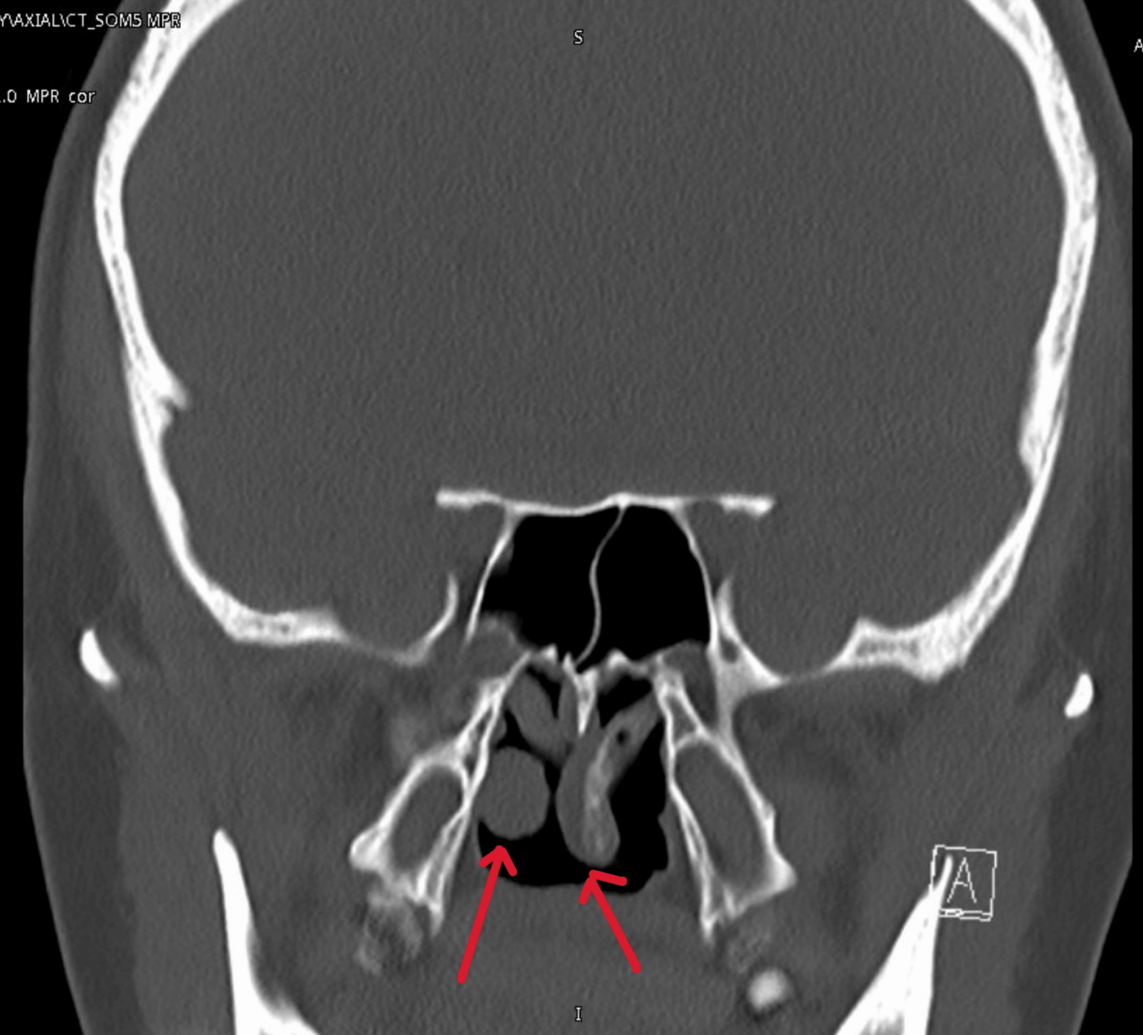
Postoperative CT scan with hanging middle turbinates in the nasopharynx
